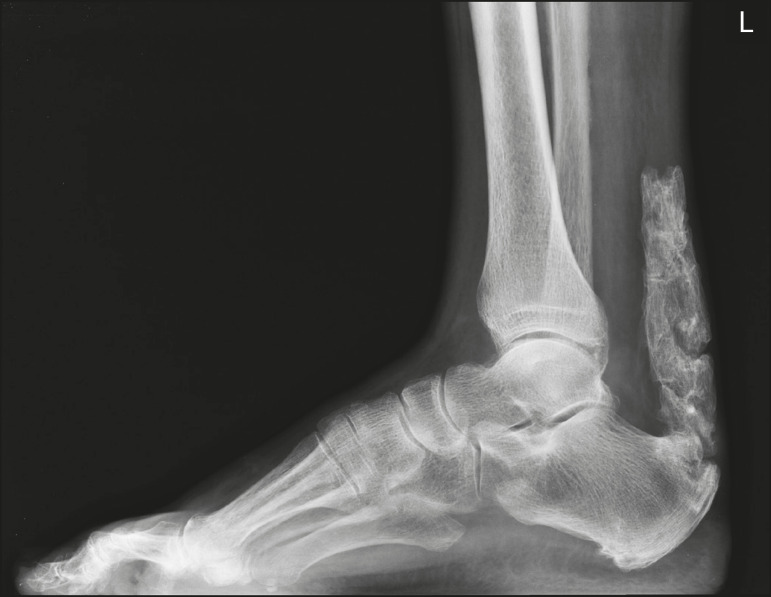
Ossification of the calcaneal tendon. Lateral X-ray of the ankle showing ossification of the calcaneal tendon after surgery to repair a tear, with an organization typical of mature bone (cortical and medullary spaces).
